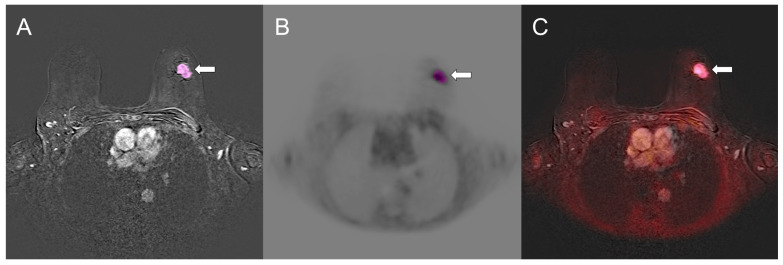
Volume of interest (VOI) placement in a 79-year-old woman with an ER-positive, G2, luminal B-like invasive ductal carcinoma (ER 80%, PR 30%, HER2 negative, Ki-67 40%) in the left breast (white arrows). (A) Semiautomated segmentation was performed on postcontrast subtracted dynamic contrast-enhanced MRI to generate a 3D-VOI encompassing the enhancing mass with indistinct borders (pink). (B) Co-registered ^18^F-FES PET images and (C) fused PET/MRI images show marked radiotracer uptake corresponding to the enhancing lesion. The VOI defined on DCE-MRI was applied to the co-registered PET dataset for extraction of quantitative PET metrics (SUVmax 4.22; SUVmean 1.83).
